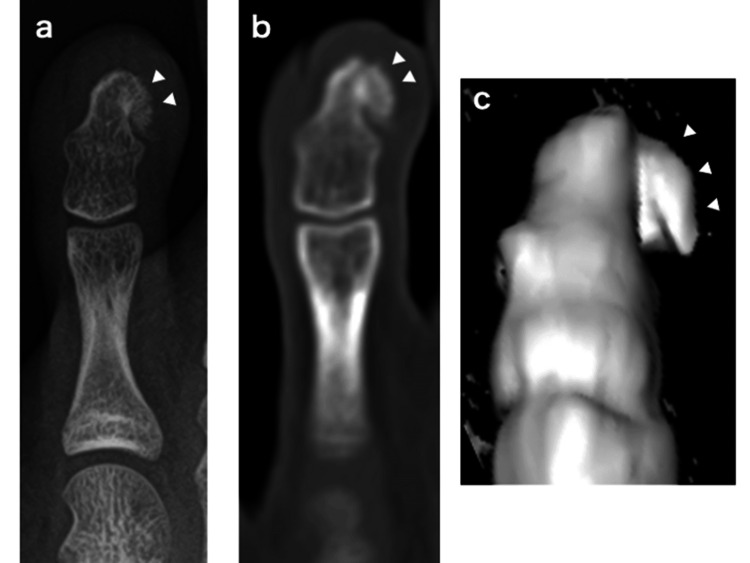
Pre-operative X-ray (a), CT (b), and 3DCT (c) images. The arrow heads indicate the tumor.
